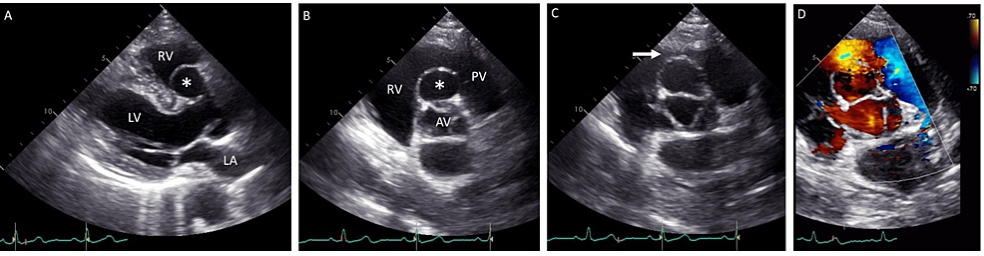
Echocardiography An echocardiographic examination reveals a sac-like structure in the right sinus of Valsalva on the parasternal long-axis view (A: asterisk) and its protrusion into the outflow tract of the right ventricle (RV) on the short-axis view at the aortic valve (AV) level (B: asterisk). Note the presence of obstruction at end-systole (C: arrow) and a mosaic pattern on color Doppler imaging (D) LA, left atrium; LV, left ventricle; PV, pulmonary valve
